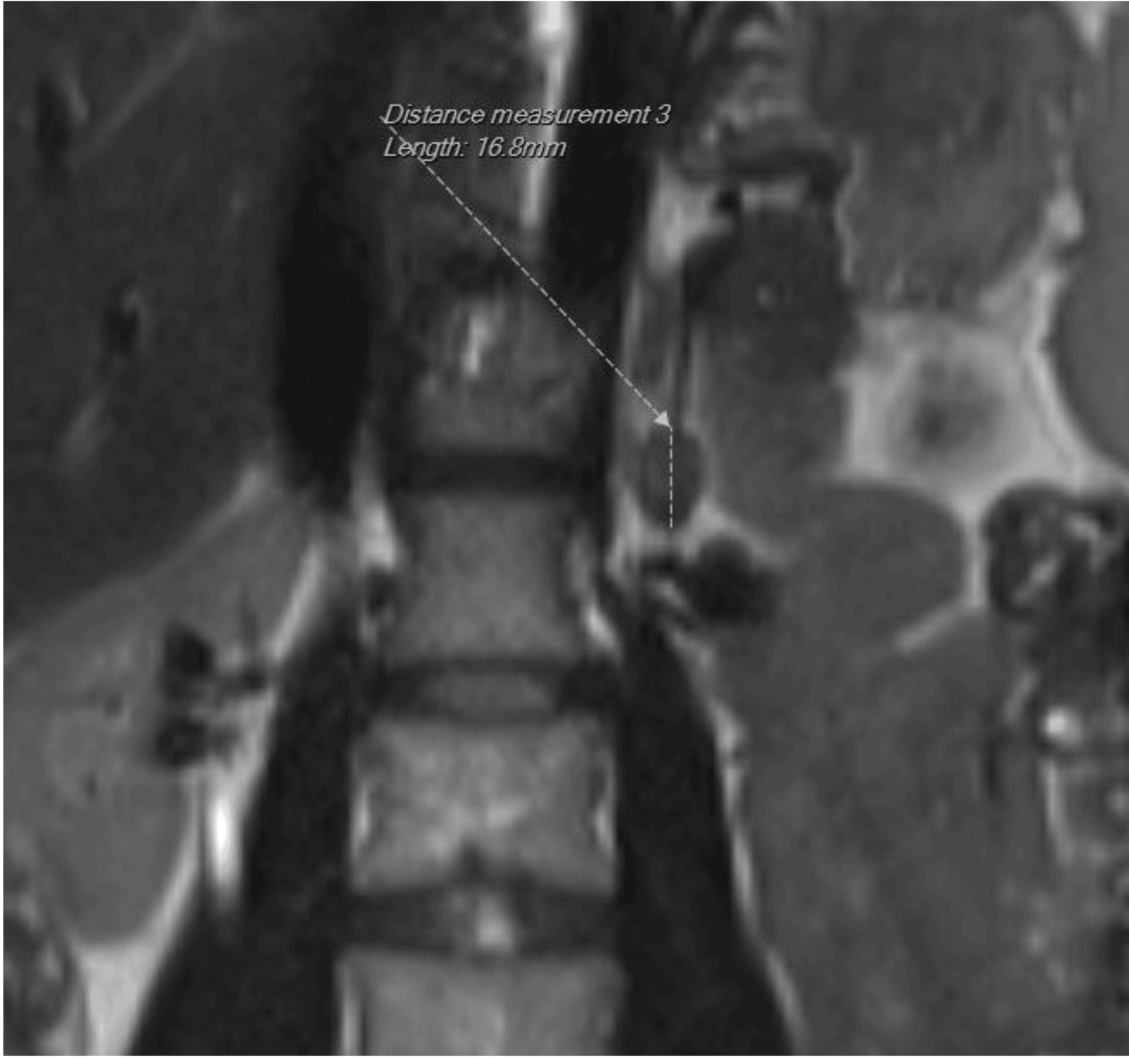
Left adrenal adenoma on MRI. Coronal adrenal-protocol magnetic resonance imaging (MRI) shows a 1.7-cm nodule in the left adrenal gland (dashed caliper, measured 16.8 mm). Adrenal venous sampling subsequently lateralized aldosterone secretion to the left, consistent with an aldosterone-producing adenoma.
